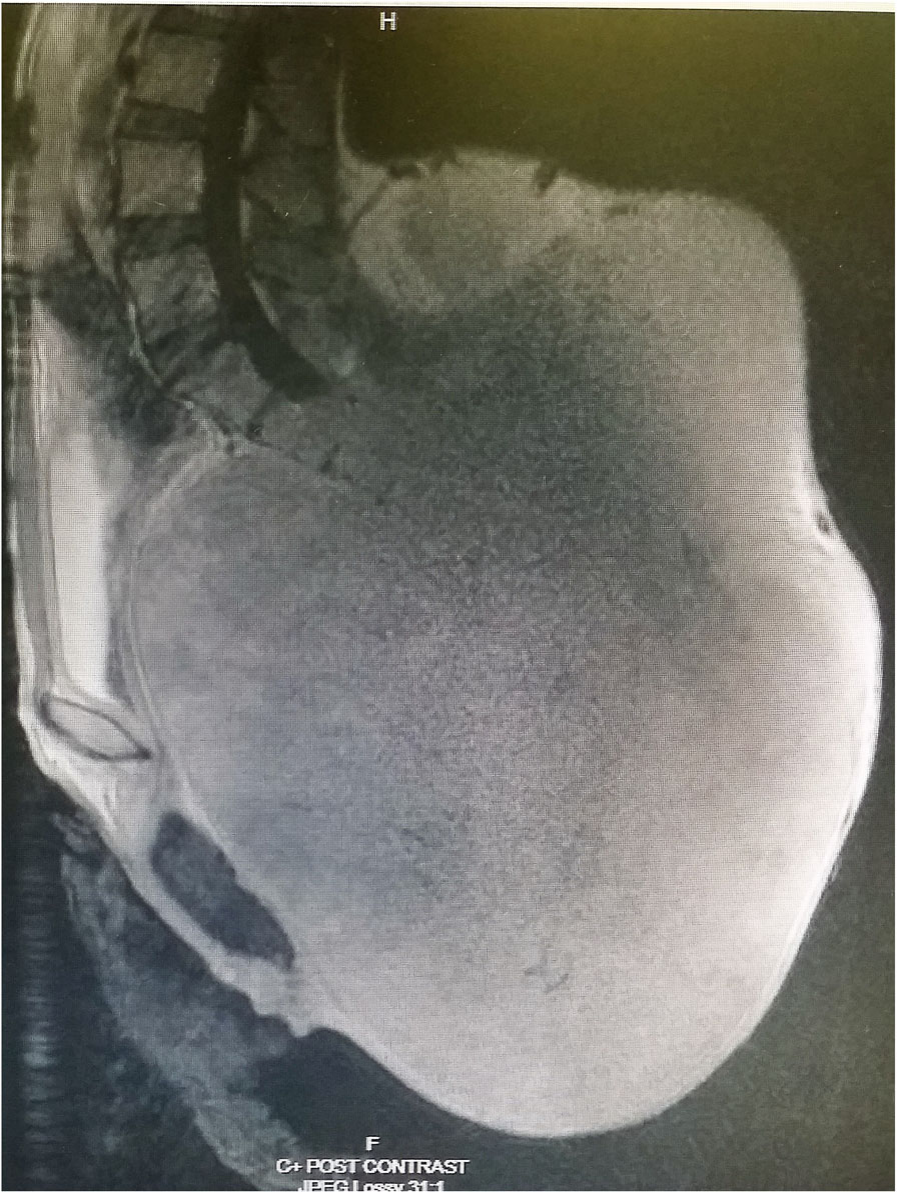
Sagittal view Computed Tomography Scan of Pelvis
